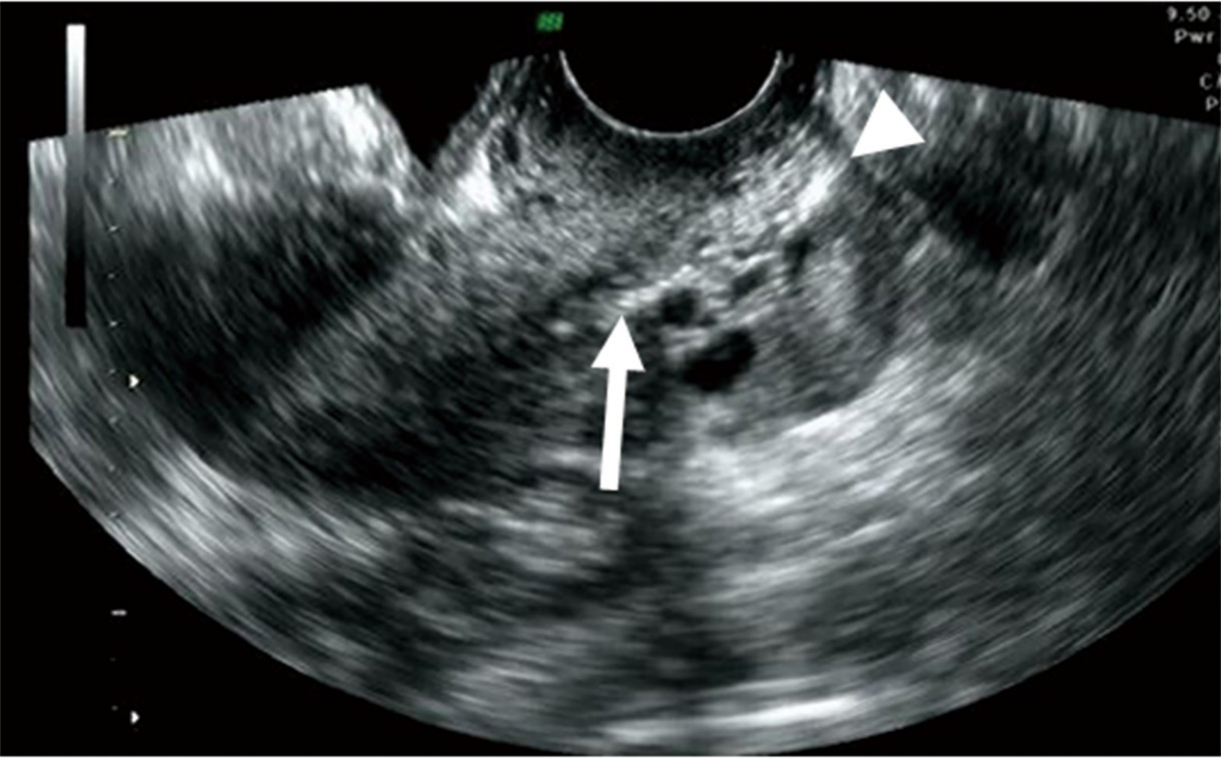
Targeted endocervical sampling. The hyperechoic line indicates the site of cytologic sampling in the cervical canal (arrow head, external cervical os; arrow, upper end of the sampling site) (transvaginal sonography).
